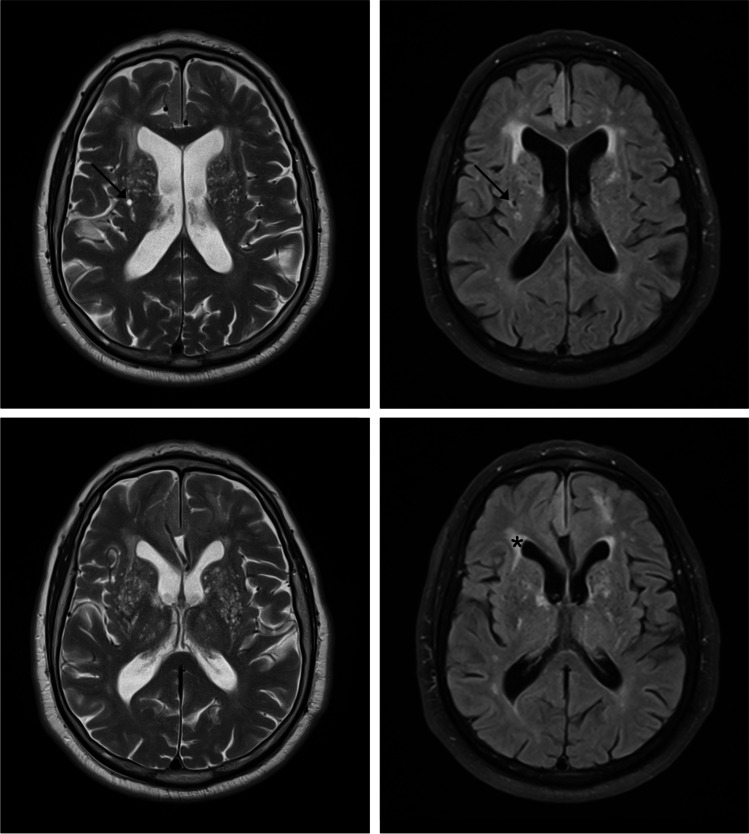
État criblé, lacunar infarct, and white matter hyperintensities. A 77-year-old man with vascular risk factors and cognitive impairment (clinically expressed as lack of executive functioning and apathy) underwent magnetic resonance imaging (MRI). T2-weighted MRI (left column) shows multiple enlarged perivascular spaces (PVS) in the form of *état criblé* at the basal ganglia. Both arrows indicate the concomitance of a small right putaminal lacunar infarct. Please note the irregularly shaped peripheral rim of gliosis hyperintense on T2-weighted fluid-attenuated inversion recovery (FLAIR) image (right arrow) helping to differentiate the lacunar infarct from adjacent PVS. In addition, please note the associated white matter hyperintensities (e.g. asterisk on the bottom right T2-weighted FLAIR image)
